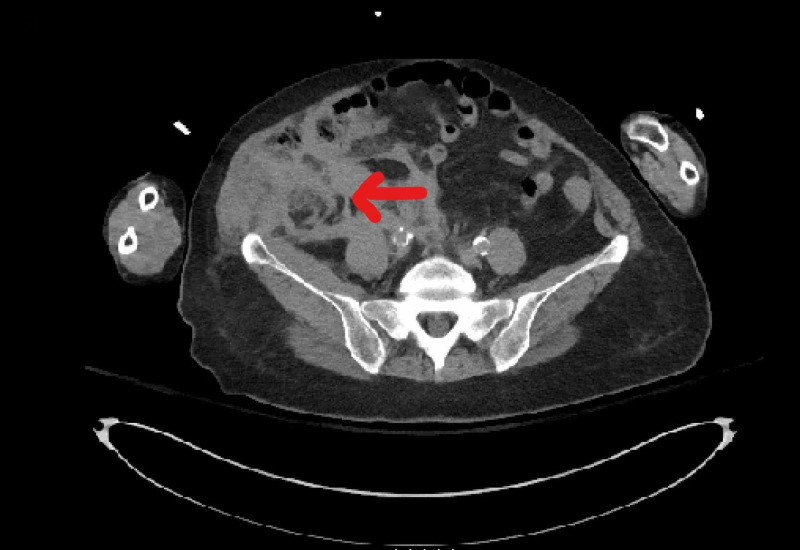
CT image with moderate right-sided retroperitoneal hematoma (red arrow) along the plane of right iliac artery
